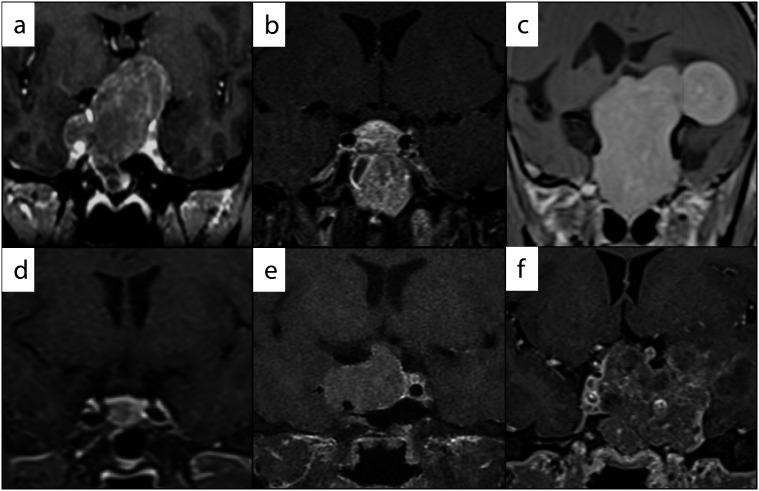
Preoperative contrast-enhanced MRI images of pituitary adenomas in patients (P) with gonadotropinomas and TSHomas. (a) P1: 30 × 23 × 42 mm with suprasellar extension; (b) P2: 34 × 28 × 29 mm, with predominant infrasellar extension; (c) P4: 42 × 37 × 60 mm, giant tumor extending into the left temporal lobe, compressing the lateral ventricles; (d) P7: 10 × 11 × 13 mm, tumor confined to the sella; (e) P8: 32 × 26 × 20 mm, predominant right parasellar extension; (f) P11: 44 × 60 × 40 mm, heterogenous tumor extending into the left temporal lobe and infrasellar extension.
